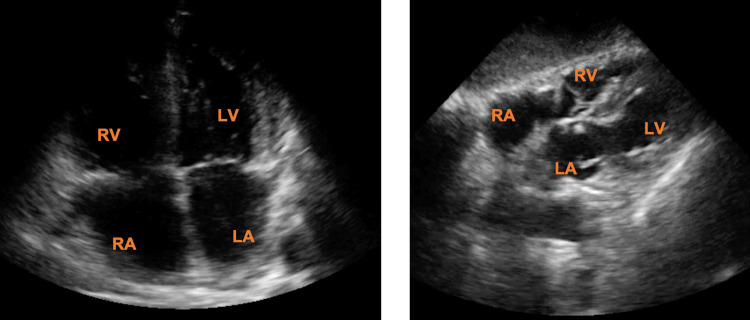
Apical four-chamber (left) and subcostal four-chamber (right) windows in two-dimensional mode. RA: right atrium; LA: left atrium; RV: right ventricle; LV: left ventricle.
